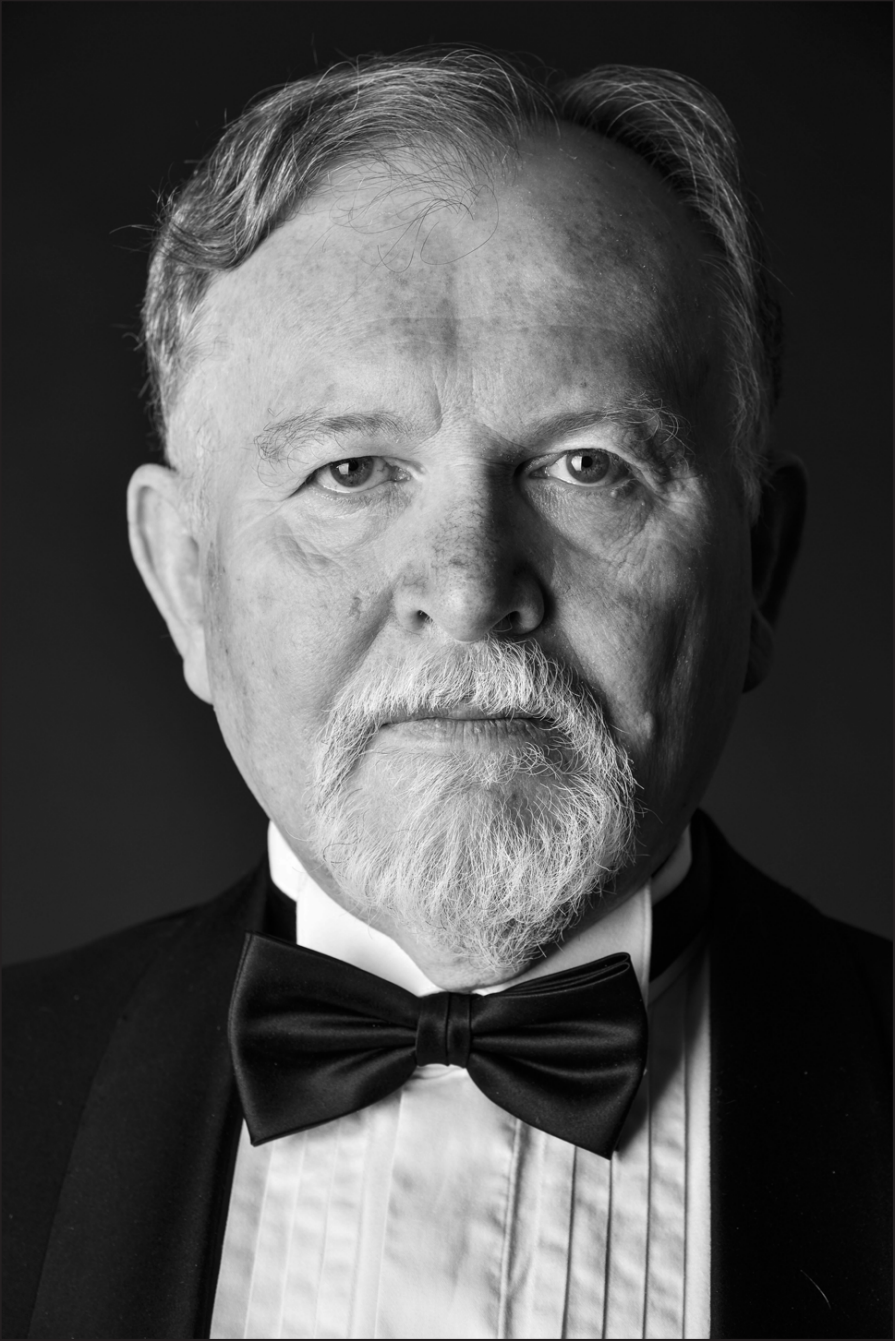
Prof. Dr. Robert F. Dondelinger (MD, PhD, Hon.FRCR (London)), graduated from University of Montpellier, France. After accomplishment of medical studies and residency training in radiology, he defended an inaugural doctorate thesis in France on ‘Abdominal Computed Tomography’ in 1978, earning the title of laureate of the Faculty of Medicine. During the 1980s, being in charge of visceral imaging at Centre Hospitalier of Luxembourg, Grand-Duchy of Luxembourg, he pioneered radiological interventions and authored articles and books in interventional techniques. In 1991, Robert F. Dondelinger was nominated full professor of Radiology at University of Liège, Belgium, and head of the Department of Medical Imaging of University Hospital Sart Tilman. He continued clinical research in vascular and visceral interventions and committed himself to international teaching. He produced many articles, contributed and edited books from 1991 to 2009, promoting imaging guided interventions. Professor Dondelinger was a member of European College of Angiography (ECA), founding member, secretary, and treasurer of the Cardiovascular and Interventional Society of Europe (CIRSE), founding president of the European Society of Thoracic Imaging (ESTI), founding member, president and gold medallist of the European Society of Gastrointestinal and Abdominal Radiology (ESGAR), president of the Royal Belgian Society of Gastroenterology (RGSGE), president of the Royal Belgian Society of Radiology (SRBR) and honorary member of other national or international scientific societies. At present, he is a consultant at the Department of Medical Imaging of University Hospital Sart Tilman, Liège.
